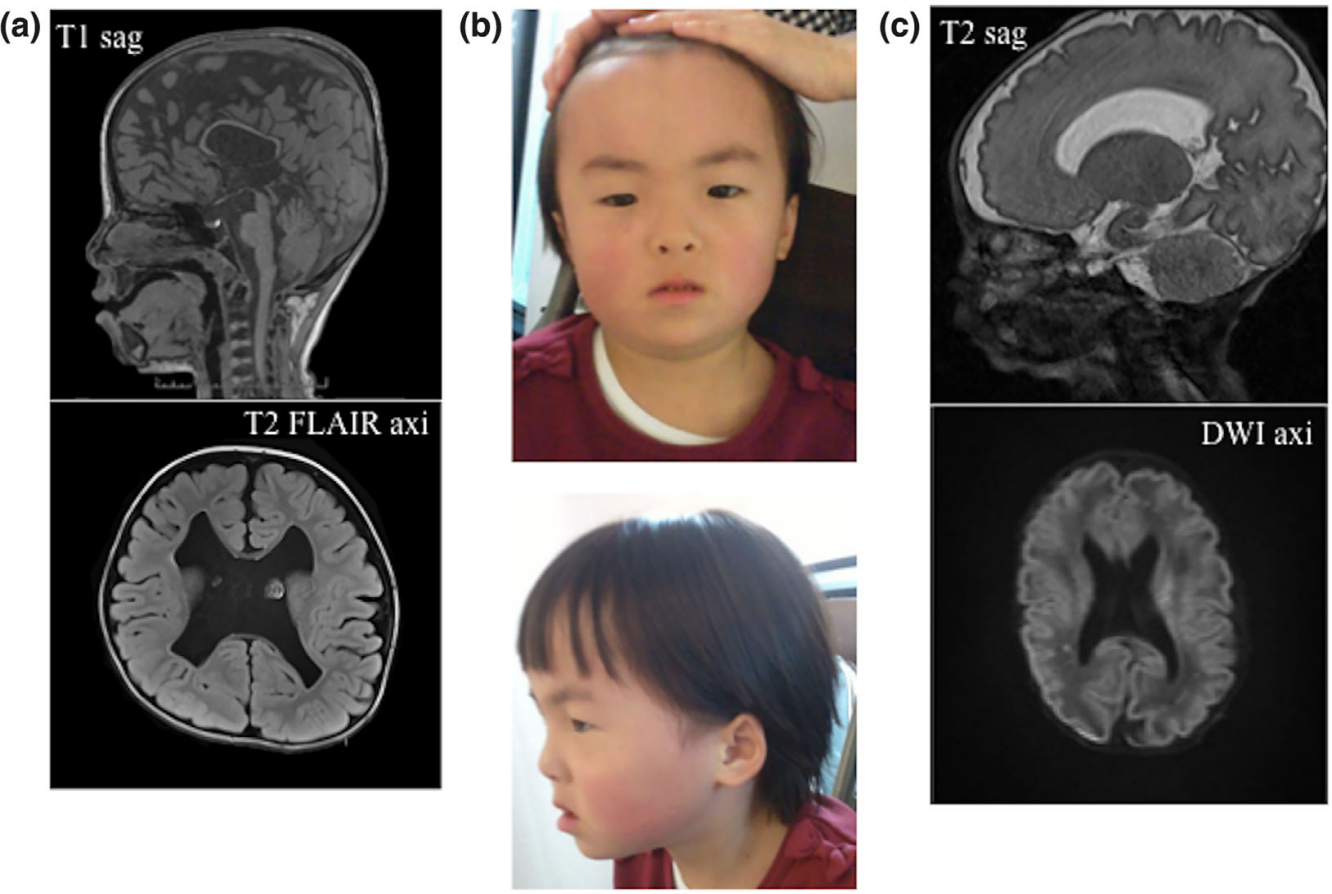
Clinical characteristics of two patients with the same *NFIA* variant. (a) Results of head MRI of Patient 1 at 5 years of age. The picture above shows sagittal T1‐weighted image. The picture below shows axial T2‐weighted fluid‐attenuated inversion recovery image. Note thin corpus callosum, cyst of septi pellucidi, ventricular wall irregularity, and decreased white matter volume. (b) Pictures of Patient 1 at 6 years of age. Note the high hairline, small eyes, anteverted nares, a depressed nasal bridge, a broad columella, and a thin upper‐lip. (c) Results of head MRI of Patient 2 at 1 month of age. The picture above shows sagittal‐T2‐weighted image. The picture below shows axial diffusion‐weighted image. Note polycerebral gyrus at parasylvius fissures, cortical dysplasia of bilateral cerebral hemisphere, partial myelination delay, and hypoplasia of corpus callosum [Color figure can be viewed at wileyonlinelibrary.com]
